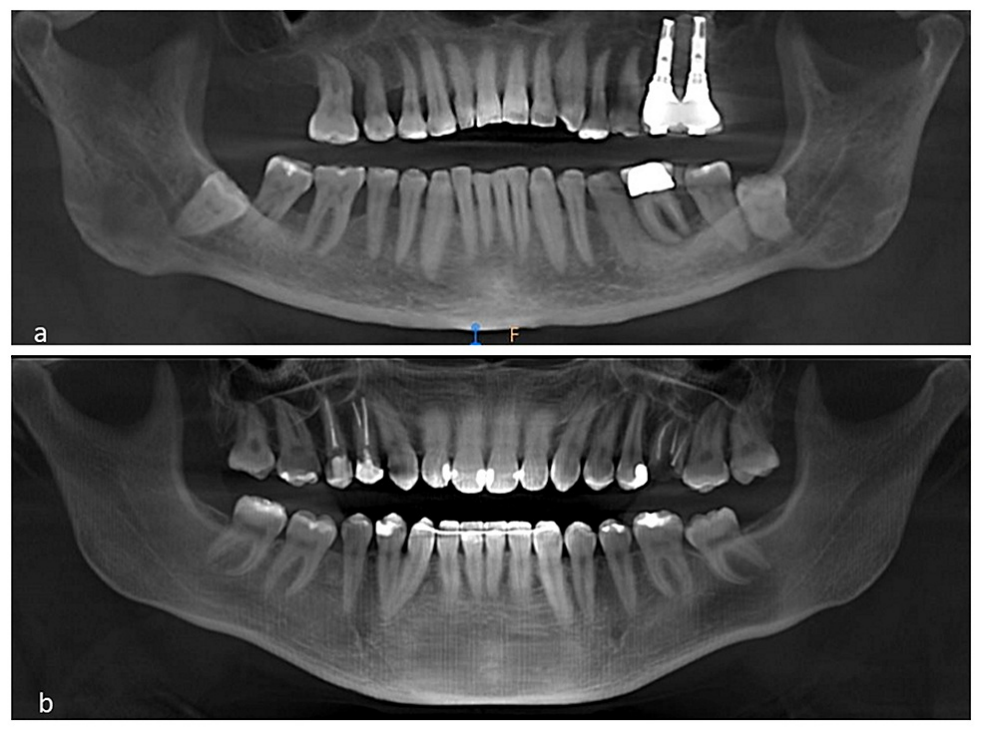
Panoramic view of the cone-beam computed tomography images a: Periodontitis case. b: Non-periodontitis case
